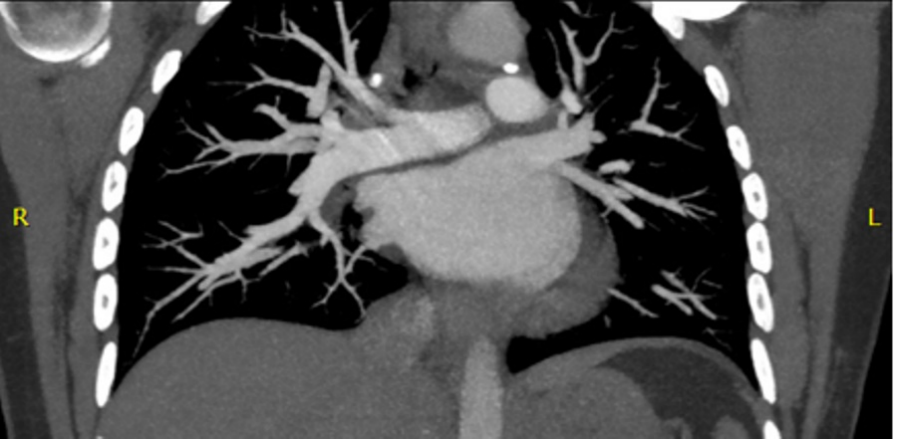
Computed tomography angiogram of pulmonary arteries showing no pulmonary embolus
